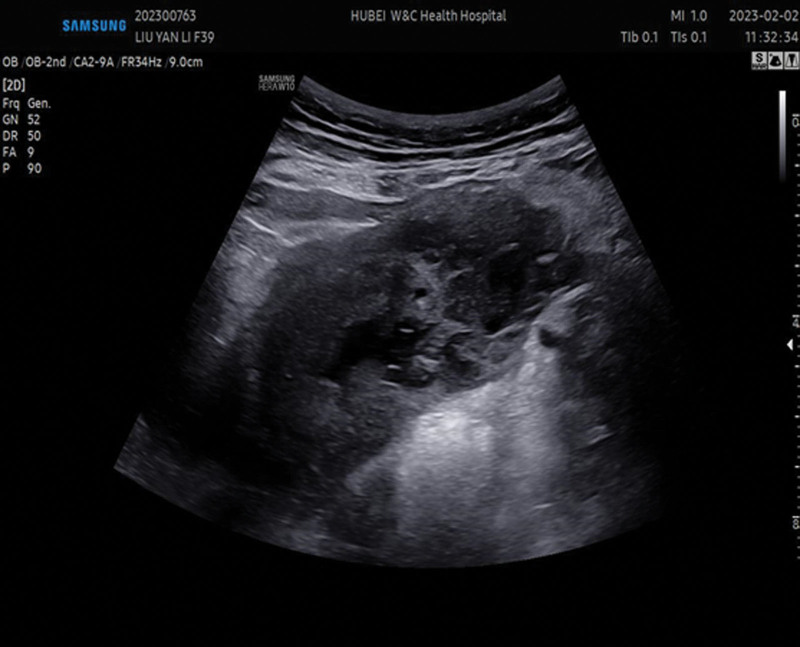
The ultrasound revealed a heterogeneous echogenic area on the lower left posterior uterine wall.
